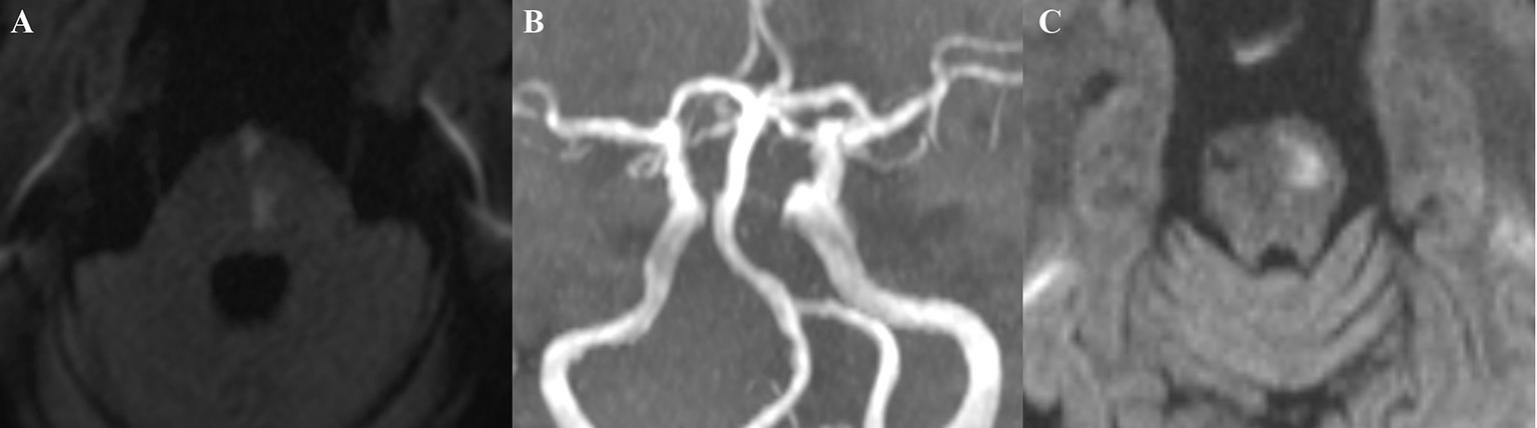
A typical case: This patient, a 69-year-old man with a history of hypertension and diabetes mellitus, was admitted to our hospital with right upper and lower extremity weakness for two days (Medical Research Council scale, grade IV). DWI showed left paramedian pontine infarction (middle pontine) (A); MRA did not reveal basilar artery stenosis, while BA dolichosis and moderate curvature were noted (B). The patient's muscle strength returned to normal after treatment. Four years later, the patient again developed weakness in the right upper and lower extremities, and a repeat DWI suggested the left basal infarction of upper pontine (C).
